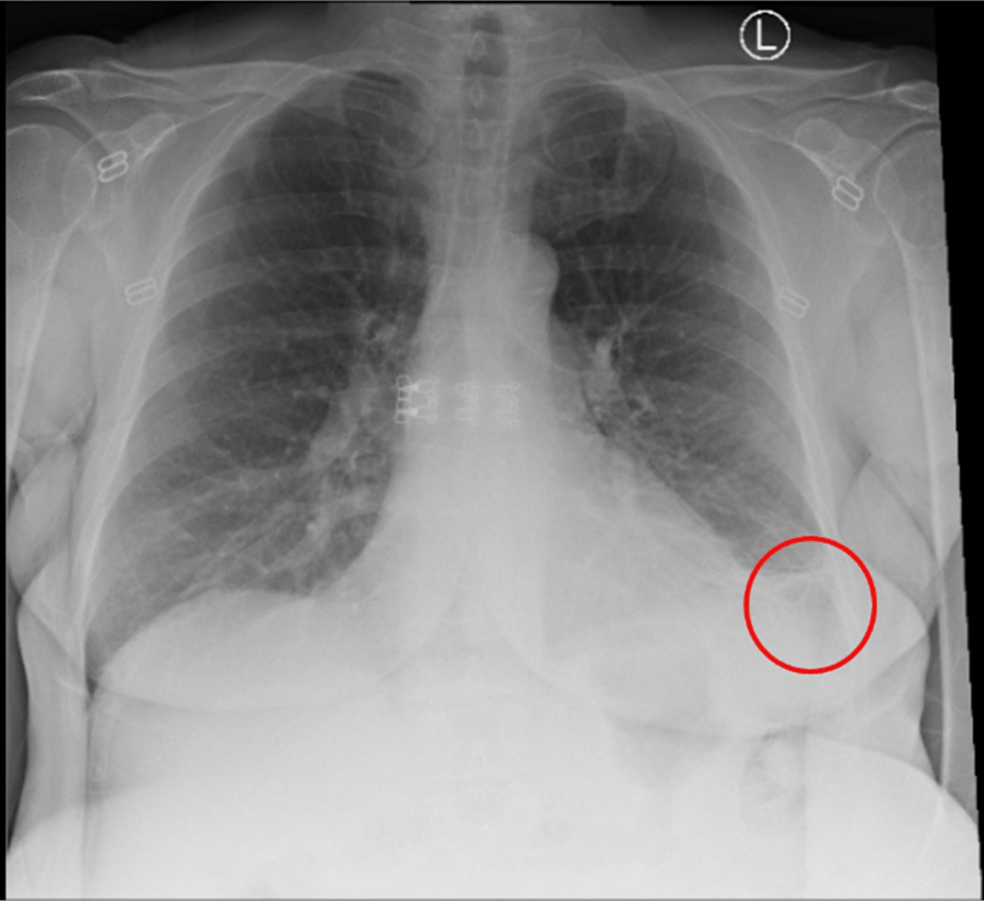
Chest x-ray showing left basilar pulmonary atelectasis vs scarring
